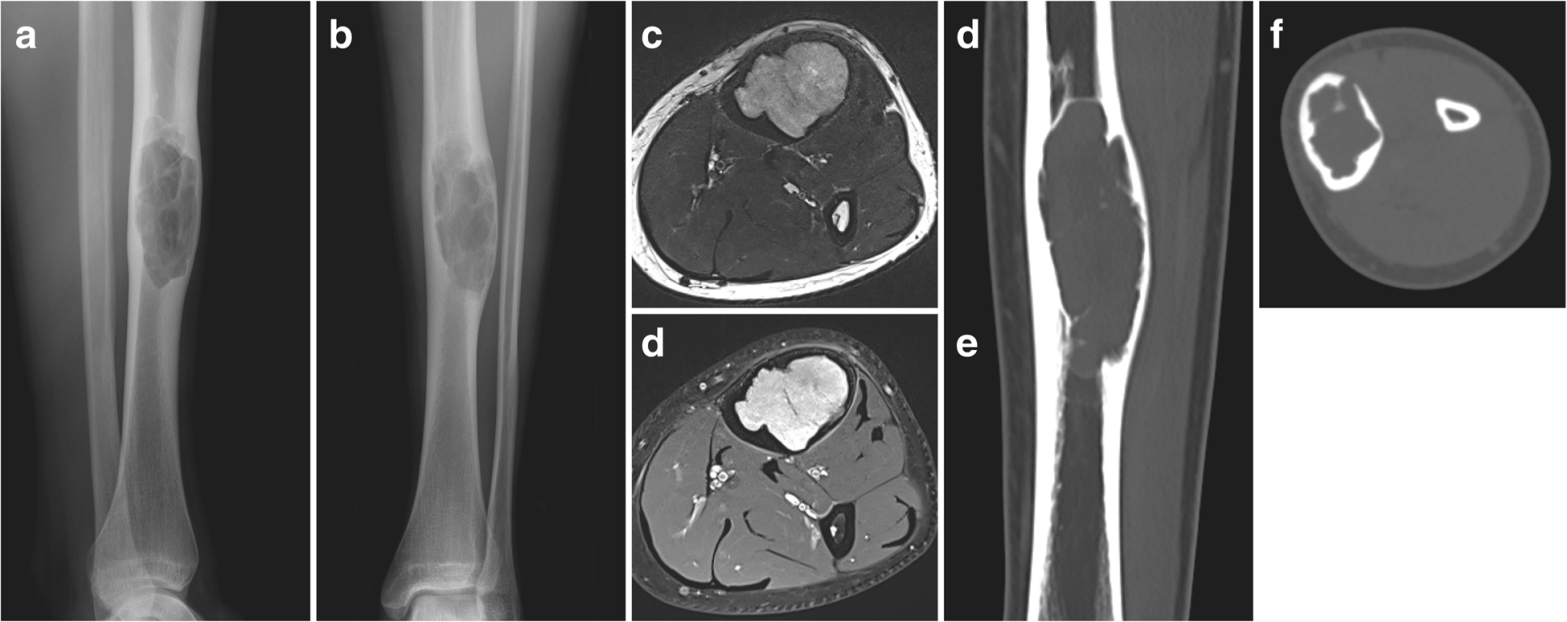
Initial imaging with radiographs (a, b), axial T2 (c) and post-contrast T1 fat-saturated dixon (d) sequences as well as CT scans (e, f) showing the lytic and lobulated aspect of the lesion in the tibial diaphysis with cortical erosion and focal penetration
